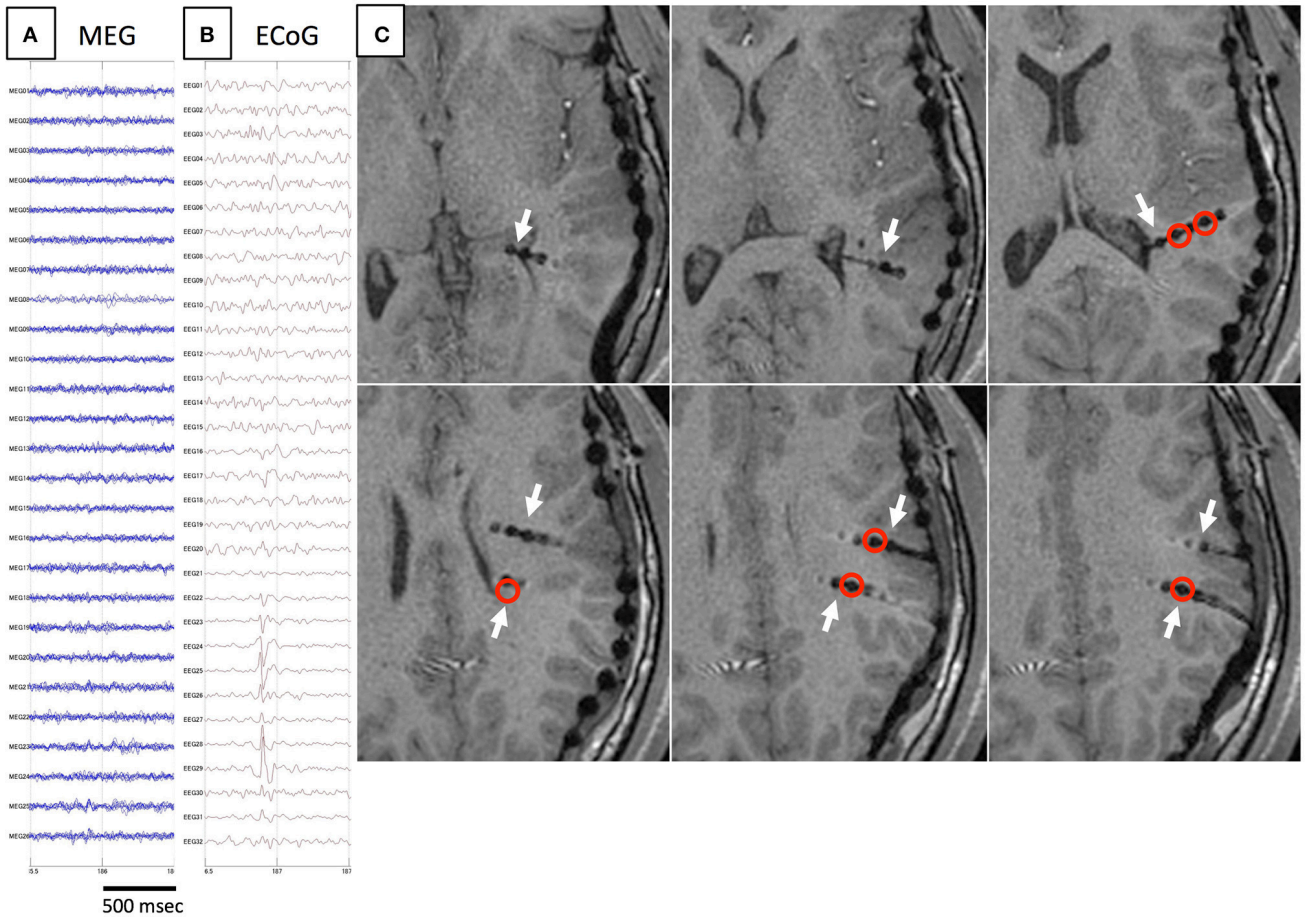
Another example of spike E. As in Figure 3, spikes are not detected on MEG (A), but only on iEEG (B). Axial MRI (C) demonstrates the location of depth electrodes (white arrows). Electrodes from which spike E are recognized are located in deep brain areas (red circles).
